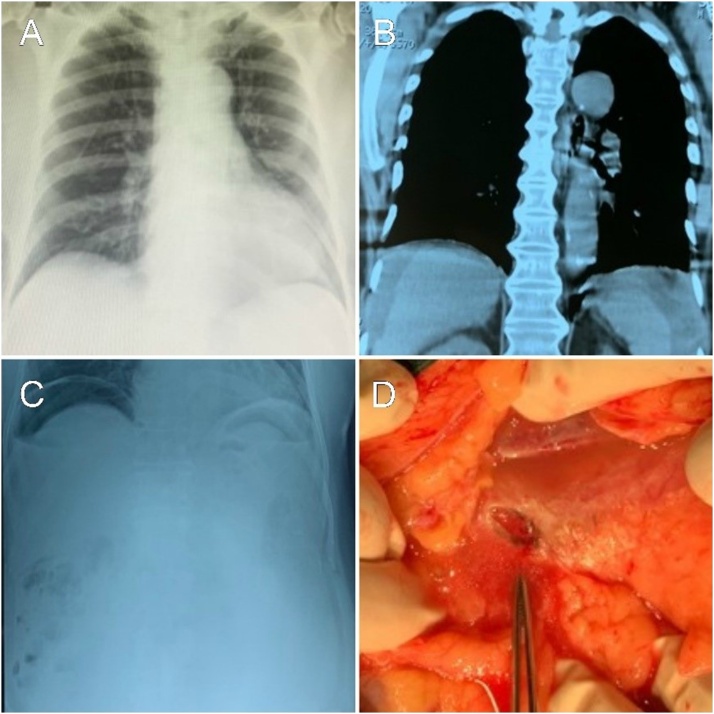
A) Chest X-ray showed bronchopneumonia, B) Chest MSCT showed emphysematous lungs, C) Abdominal X-ray showed pneumoperitoneum, and D) Intraoperative finding of gastric perforation.
